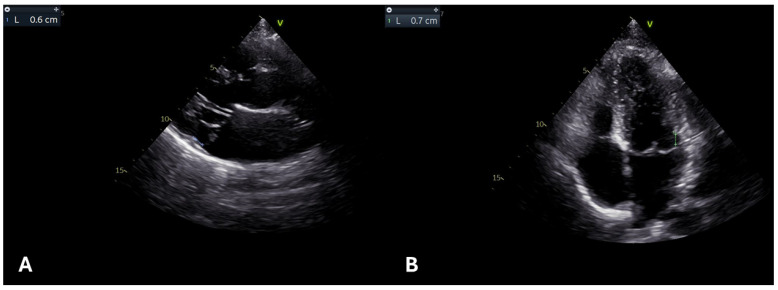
(A,B) show mitral annular disjunction (MAD) in 2D transthoracic echocardiography (TTE) of a young patient with Marfan syndrome, respectively, captured in the long parasternal view and the four-chamber view. The MAD measures 0.6 cm and 0.7 cm, respectively.
